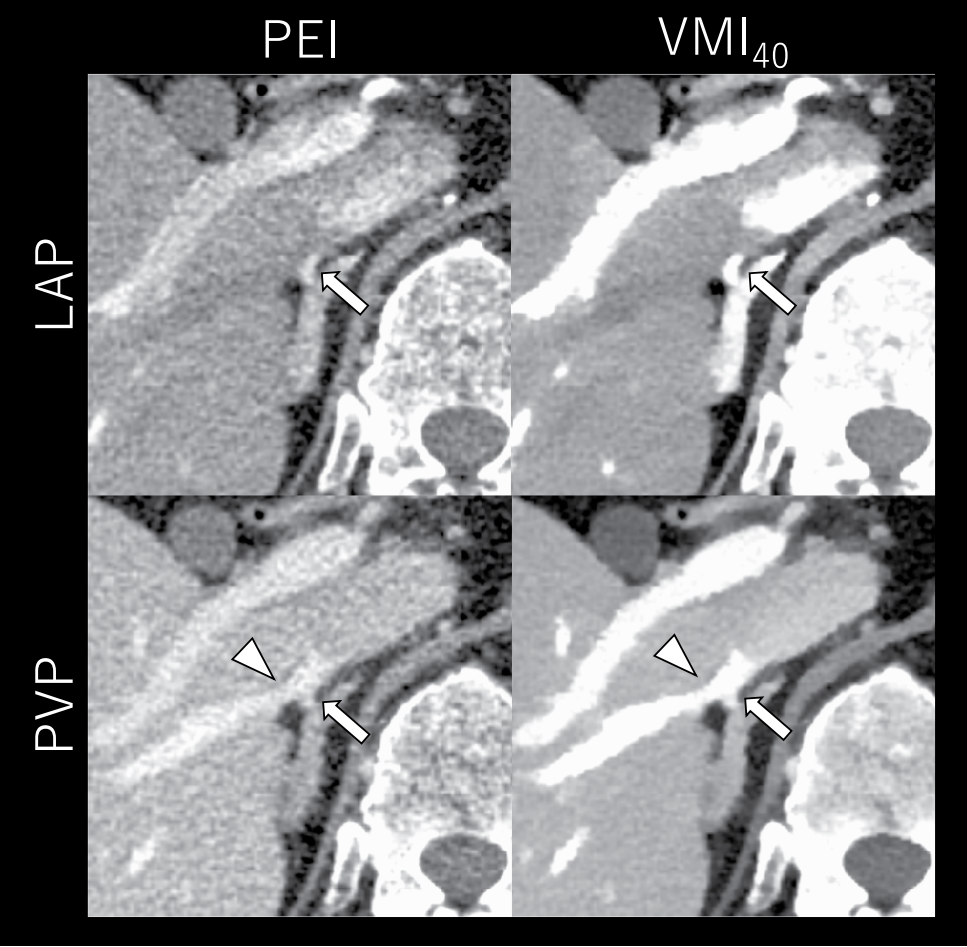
CT images of a 66-year-old male with primary aldosteronism (BMI: 26.5 kg/m^2^). The upper and lower rows display late arterial phase (LAP) and portal venous phase (PVP) images, respectively, reconstructed with polyenergetic images (PEI, left column) and virtual monoenergetic images at 40 keV (VMI_40_, right column). The window display is optimized for each image series. VMI_40_ at PVP provided a clearer depiction of RAV (arrows) than PEI at LAP and most effectively visualized its relationship with accessory hepatic vein (arrowheads), both draining into the inferior vena cava after forming a common trunk
